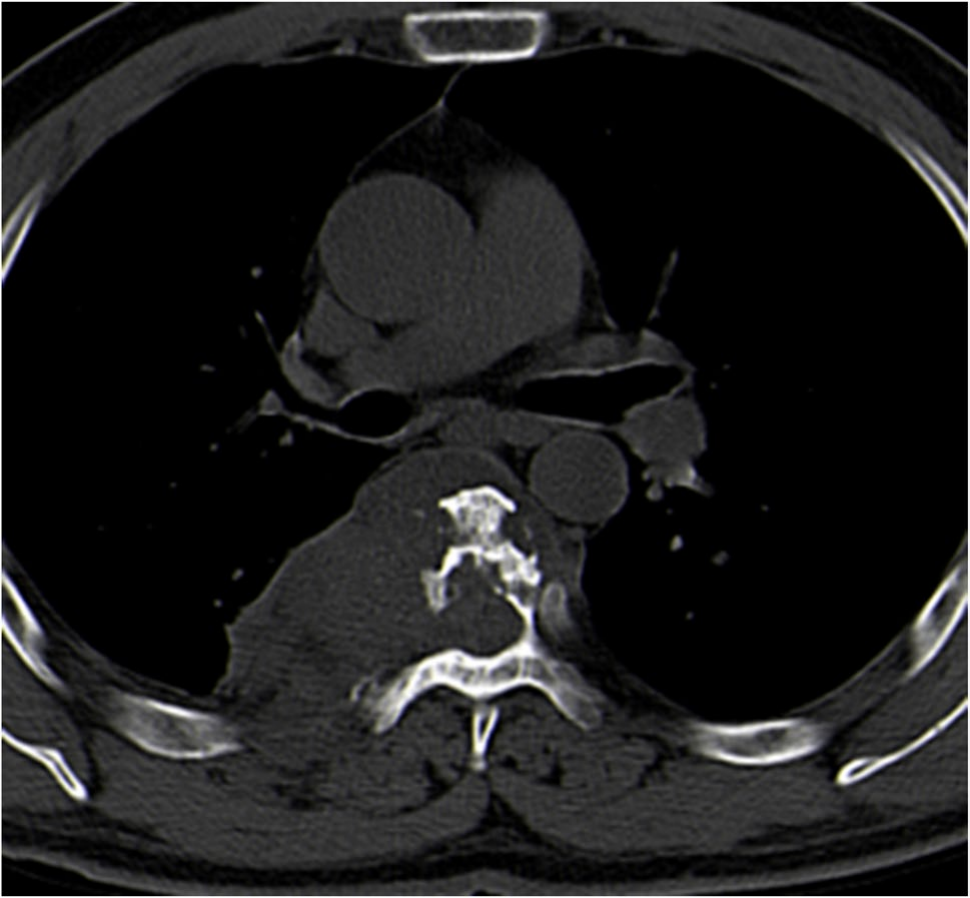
Plain CT scan revealed irregular bone destruction of the 7th thoracic vertebrae with hydatid cyst in surrounding soft tissue
